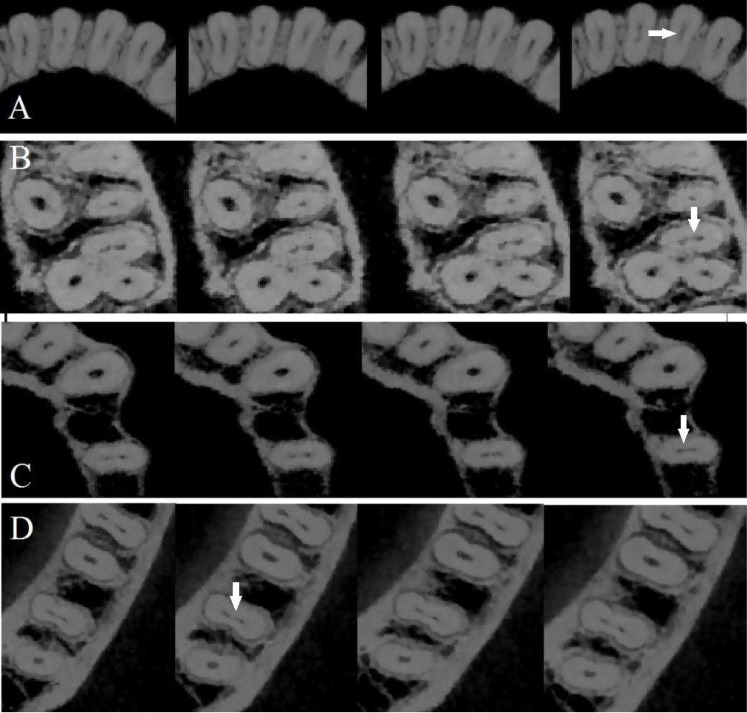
A) *Complete isthmuses in mandibular central incisor; *B)* maxillary second molar; *C)* maxillary second premolar; *D)* mandibular second molar viewed in CBCT axial slices (interval, 0.1 mm; thickness, 0.1 mm) from coronal to apical direction. Arrows indicate isthmus
